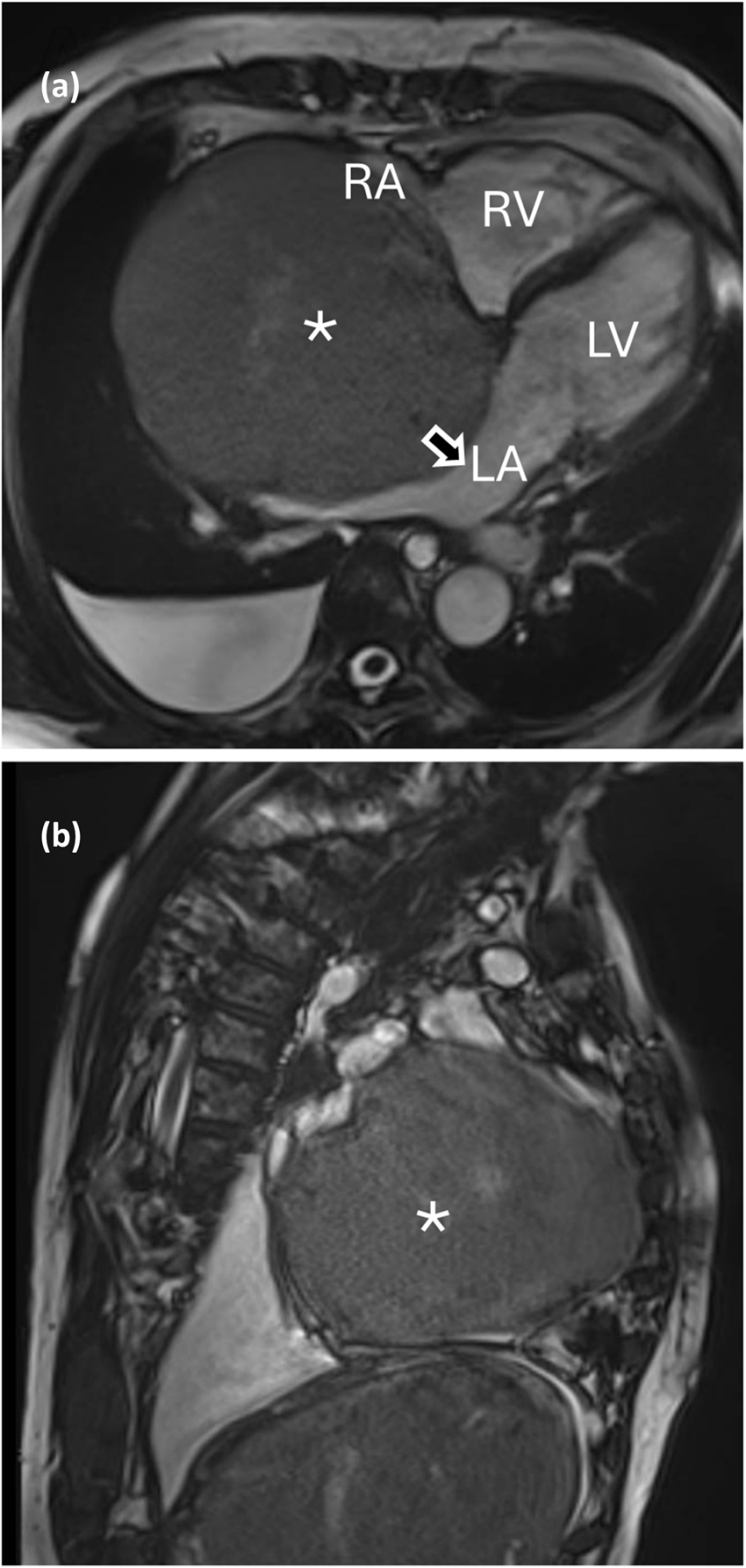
(a) and (b) Tumor in the MRI. RV: right ventricle; LV: left ventricle, LA: left atrium, RA: right atrium, arrow: interatrial septum, *: intracardiac mass.
